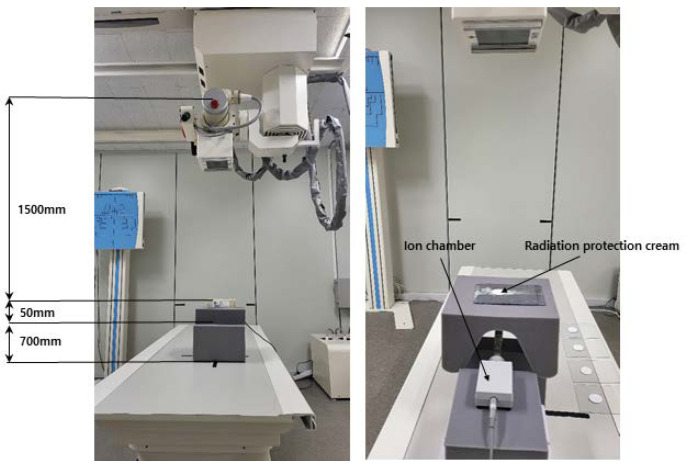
X-ray shielding performance evaluation method.
